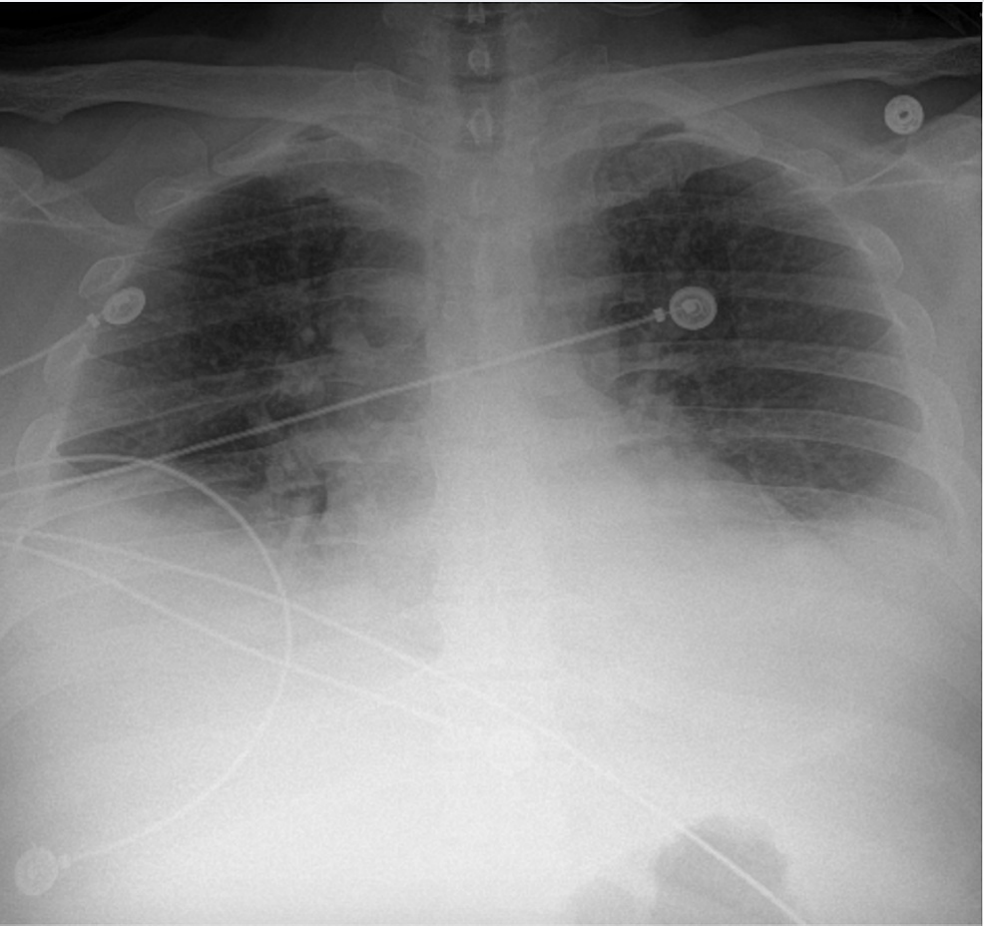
Chest X-ray showing bilateral infiltrates with blunting of the costophrenic angles.
